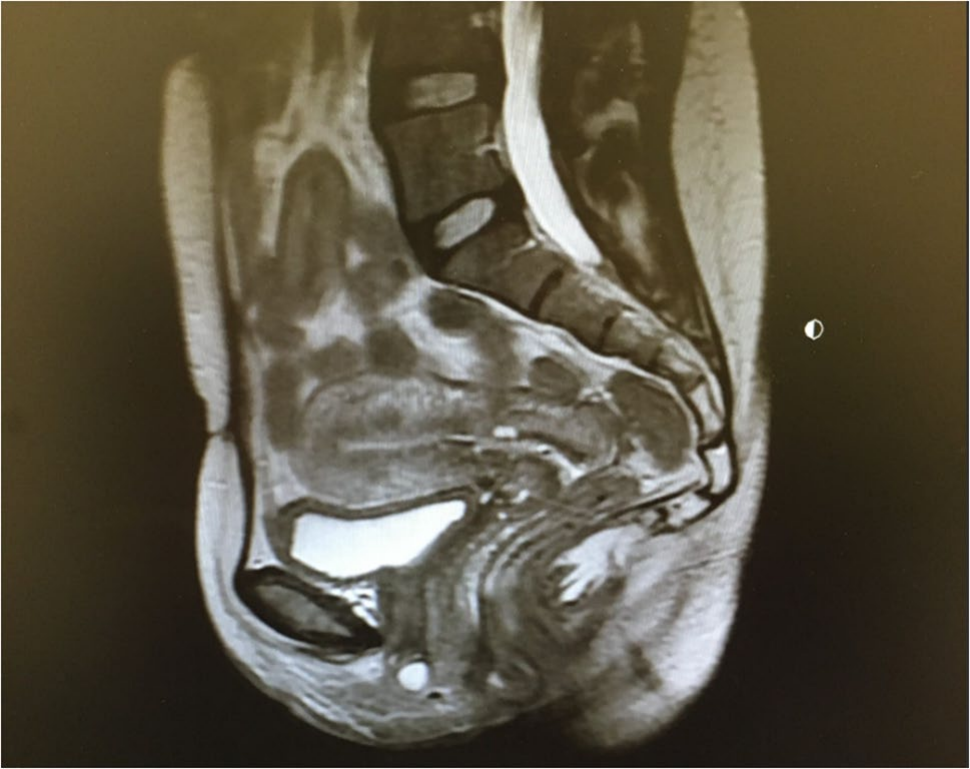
Magnetic resonance image at rest with no prolapse
